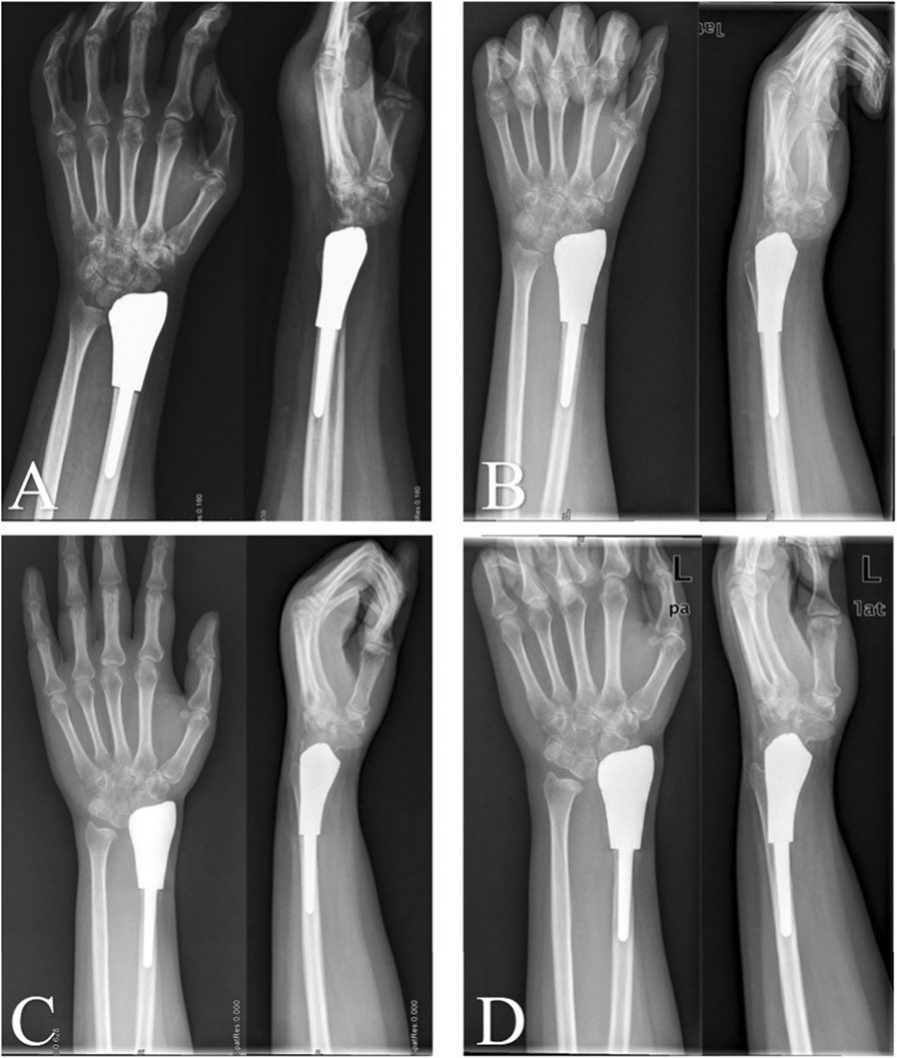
The serial X-rays show the separation of left DRUJ after prosthetic reconstruction. A: 2 days after surgery; B: 1 month after surgery; C: 12 months after surgery; D: 42 months after surgery
